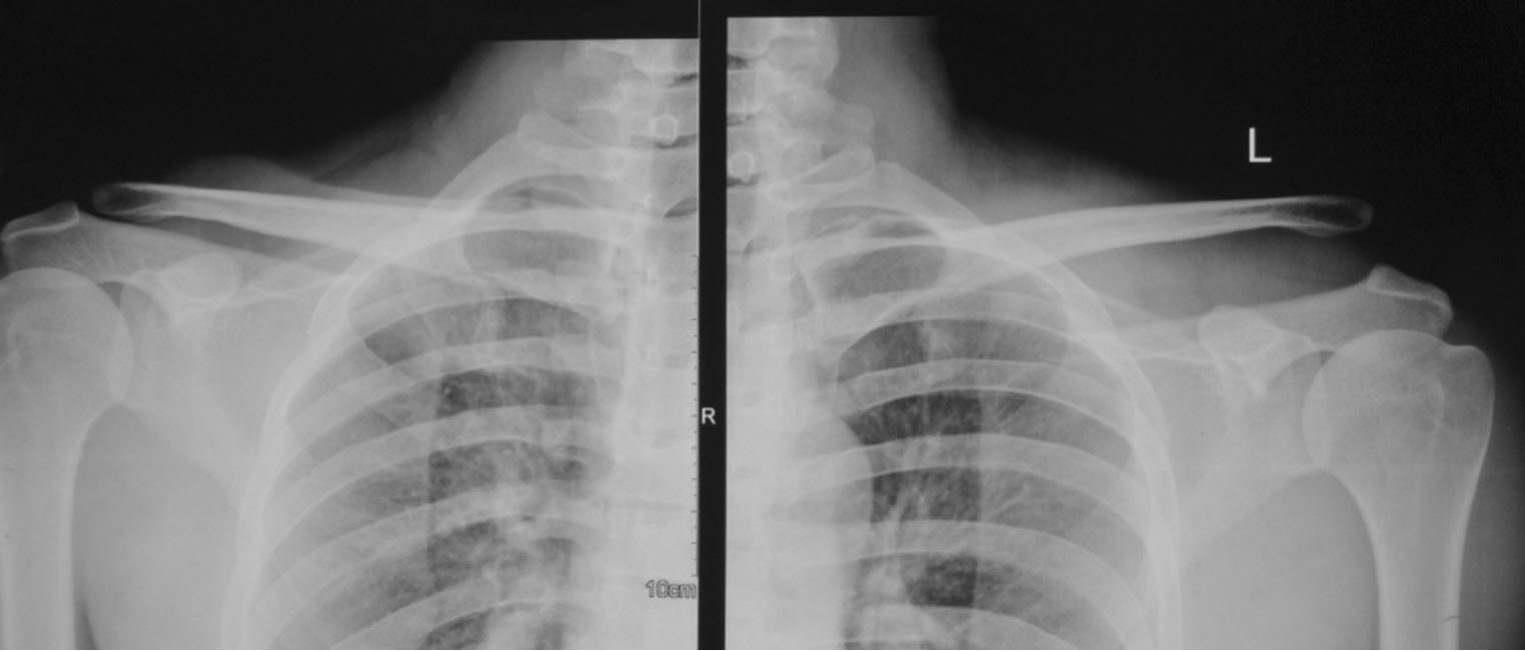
Pre-operative radiograph showing grade III acromioclavicular dislocation and an increased coracoclavicular distance
